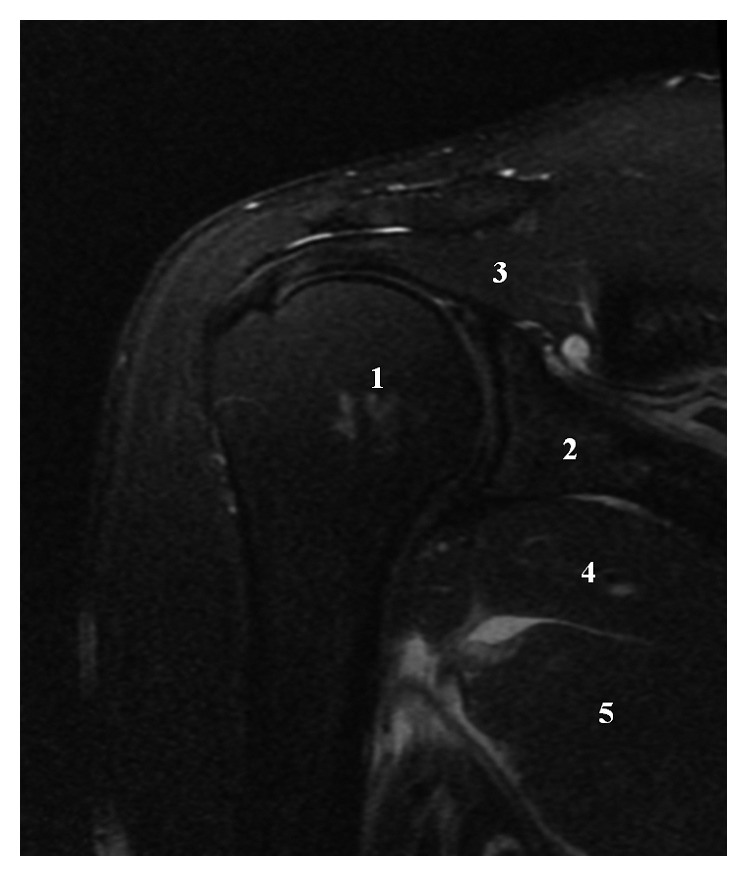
Coronal T2 MRI: (1) humeral head, (2) glenoid cavity, (3) supraspinatus muscle, (4) teres minor muscle, and (5) complete rupture of the teres major muscle.
